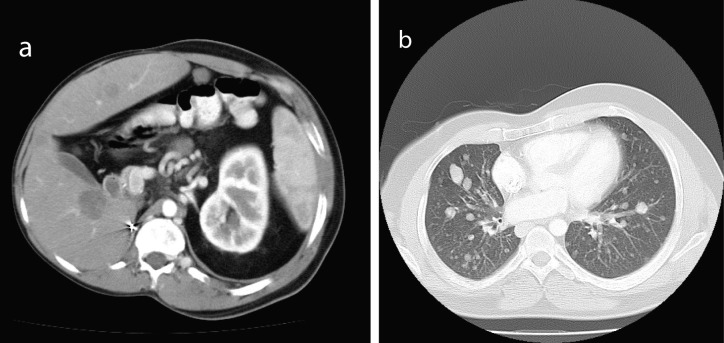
Staging CT showed multiple metastases (a, b).
